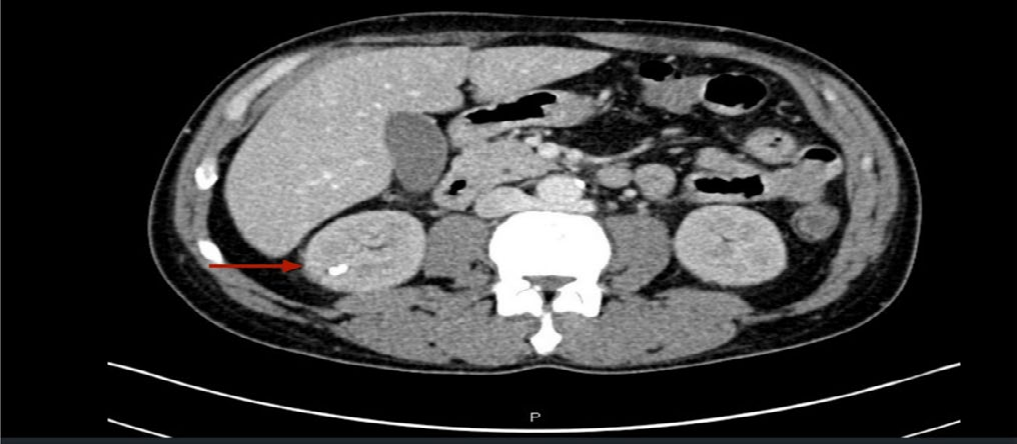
Computed tomography was unremarkable for any cortical or medullary damage.
